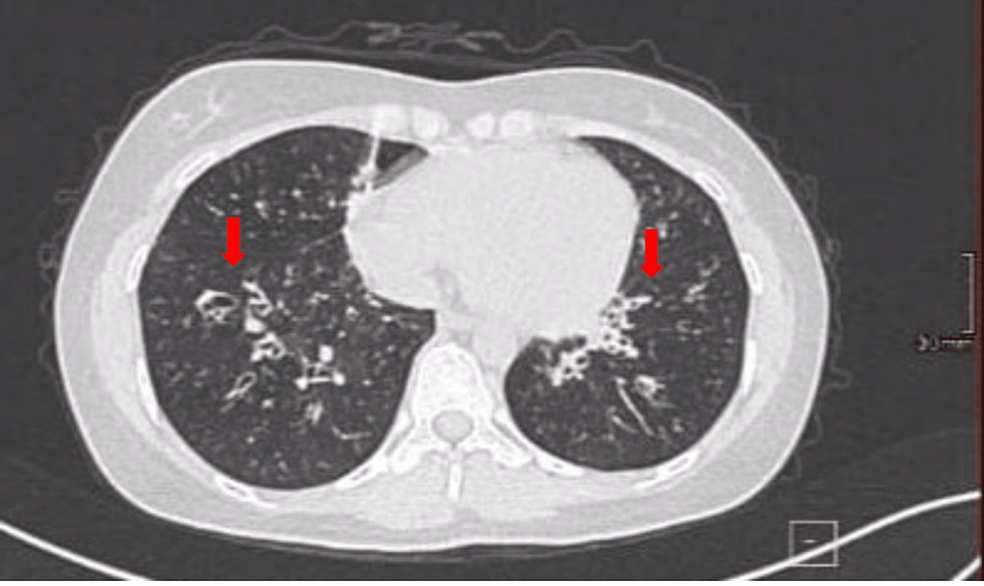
Chest CT image A chest CT image revealing central bronchiectasis (red arrows). CT, computed tomography.
